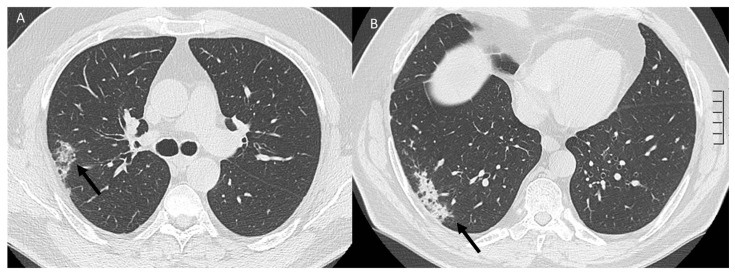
CT evaluation (A,B) of RRP induced by COVID-19 vaccine in RT-treated patients with lung metastases: consolidative pattern (arrows).
